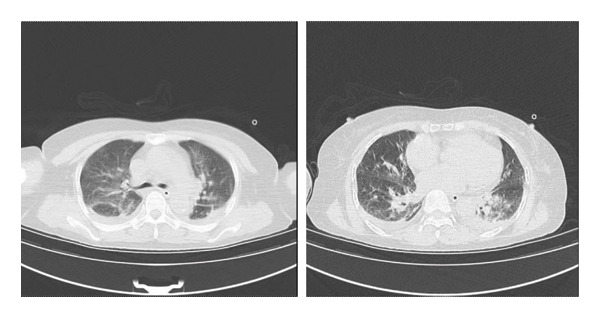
The chest CT on the 9th day after initial symptoms.
